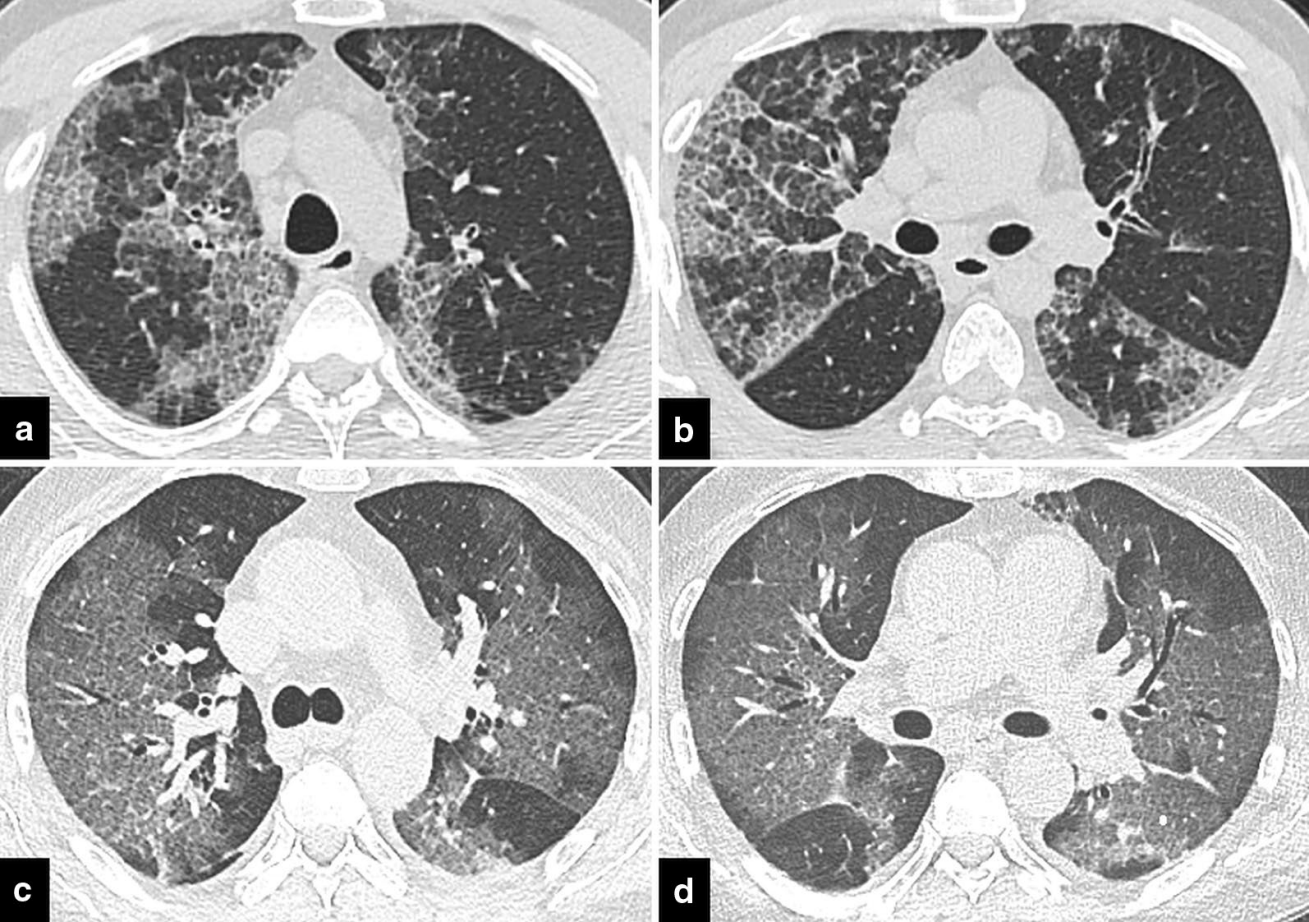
Crazy-paving pattern. a, b Crazy-paving pattern in pulmonary alveolar proteinosis (PAP). c, d Axial CT images of a patient with COVID-19 show widespread GGOs with crazy-paving pattern in both lungs
